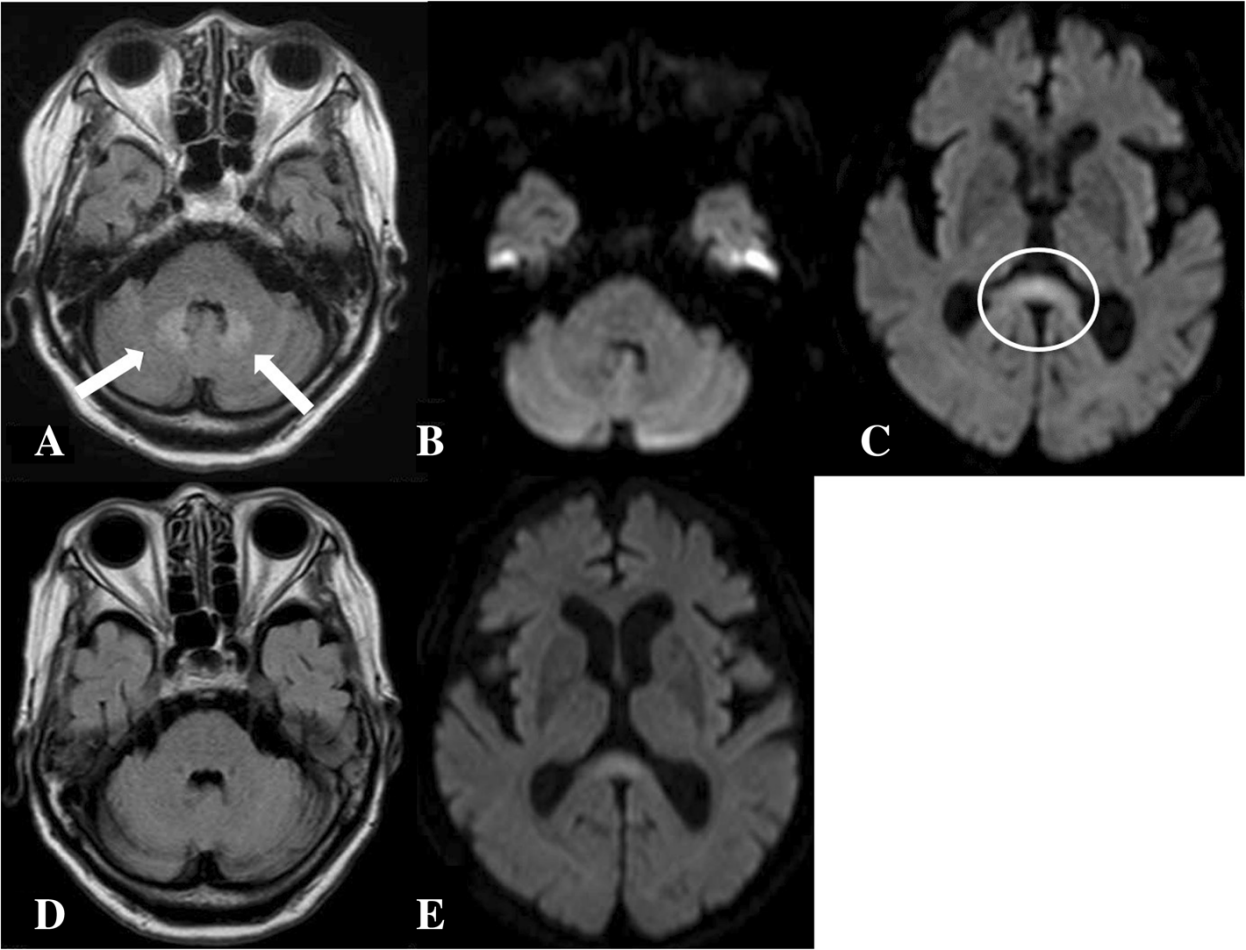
a Brain magnetic resonance imaging. T2-weighted fluid-attenuated inversion recovery (FLAIR) imaging performed at 4 days after symptom onset shows hyperintense areas in the bilateral basal dentate nuclei (white arrows). b Diffusion-weighted imaging (DWI) performed 4 days after symptom onset showed an almost normal intensity in the bilateral basal dentate nuclei. c DWI performed 4 days after symptom onset revealed a hyperintense T2 signal with compatible cytotoxic edema (white circle). d At 14 days after discontinuation of oral metronidazole, abnormal findings on T2-weighted FLAIR imaging completely disappeared. e At 14 days after discontinuation of metronidazole, the abnormal findings on DWI almost disappeared
